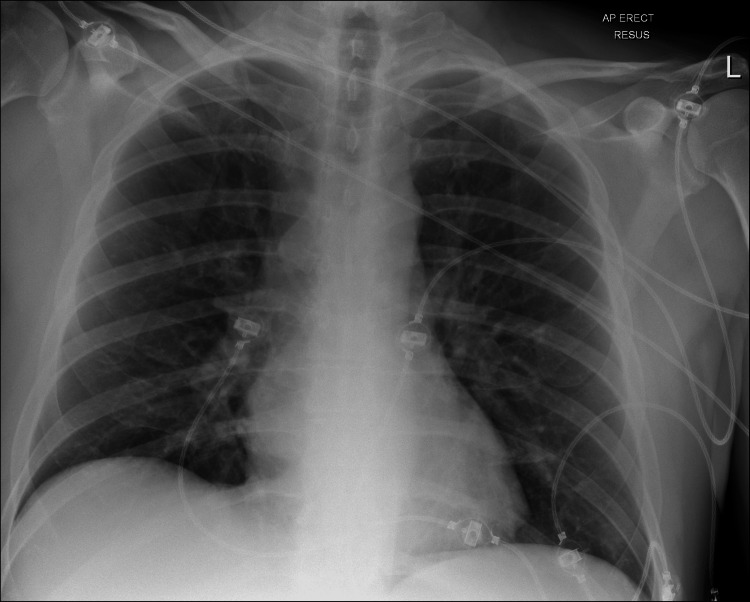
Initial chest X-ray Anterior-posterior erect view
